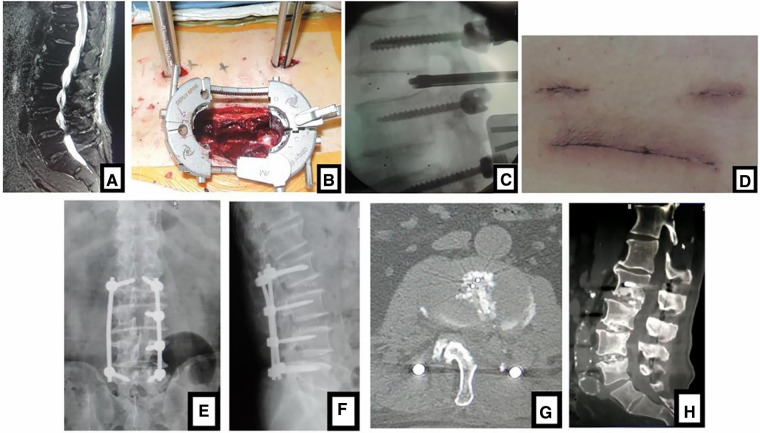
(A) sagittal MR images showed 3-level LDD (L2–L5) in a 75-year-old man with neurologic symptoms. (B) Contralateral bridge Freehand MIPS combined with unilateral MIS-TLIF through tube was performed. After the cage was inserted into the intervertebral space through the tube, (C) the fluoroscopic image confirmed the position of cage. (D) The picture was postoperative incision like smile face, so this MIS-TLIF is called smile-face surgery. (E, F) Postoperative x-ray showed that the position of pedicle screws and cages was good and (G) axial CT scan confirmed that the neurologic decompression was complete. In (H) sagittal CT at 5-year follow-up, fusion of grade I or II was achieved.
